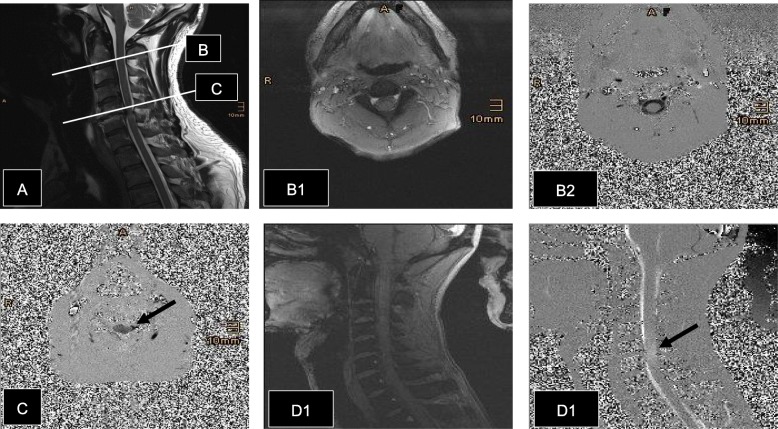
Patient with spinal cord compression at cervical level C5/6. A Sagittal T2w MRI, lines indicating axial levels C2 and C5. B1 Axial magnitude. B2 Axial phase-imaging at level C2, spinal cord and CSF-space can be is easily distinguished. The gray-level within the phase-images encode the measured velocities in cranio-caudal direction, dark gray velocities in caudal direction, light vice versa. Bright white or black are aliasing effect. The gray intensity signal of the spinal cord is similar to the surrounding neck tissues. C Axial phase-imaging at level of stenosis C5; there is barely any CSF-space left, only minor CSF-motion can be seen at the left side of the spinal cord (arrow). The gray intensity signal of the spinal cord is much darker than the surrounding neck tissues. D1 Sagittal magnitude. D2 Sagittal phase-imaging. Here, light gray signals encode cranio-caudal velocities and dark gray encodes the opposite direction. At the level of stenosis, a local increase in spinal cord motion can be observed (arrow)
